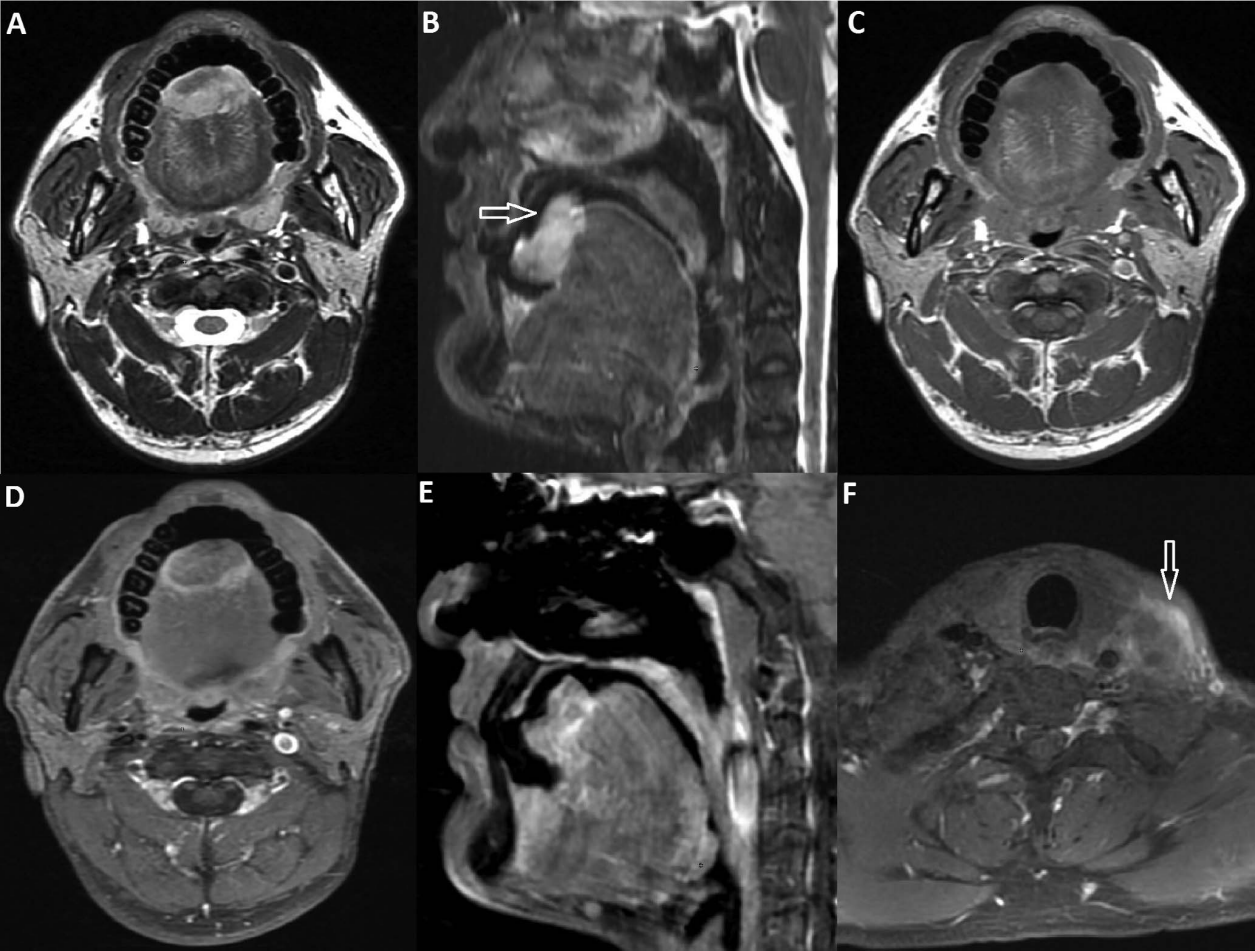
Magnetic resonance imaging (A) T2-weighted axial and (B) short TI inversion recovery sagittal images show a hyperintense, irregular, partly exophytic mass involving the anterior tongue (arrow). (C) On T1-weighted image, the mass is hypointense. Postcontrast T1-weighted (D) axial and (E) sagittal images show heterogeneous contrast enhancement. (F) Postcontrast T1-weighted axial image shows a peripherally enhancing necrotic metastatic lymph node at level 4 on the left side (arrow).
